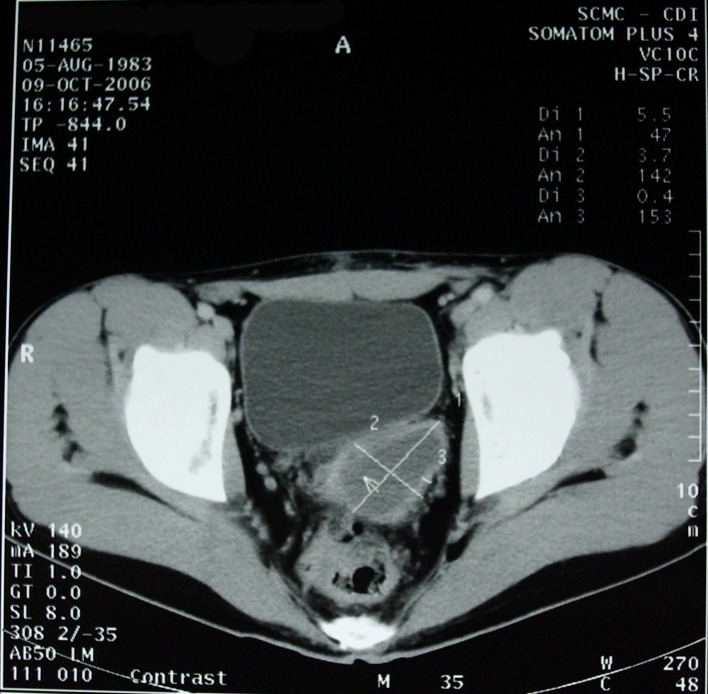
Postoperative CT scan, showing the seminal vesicle.
